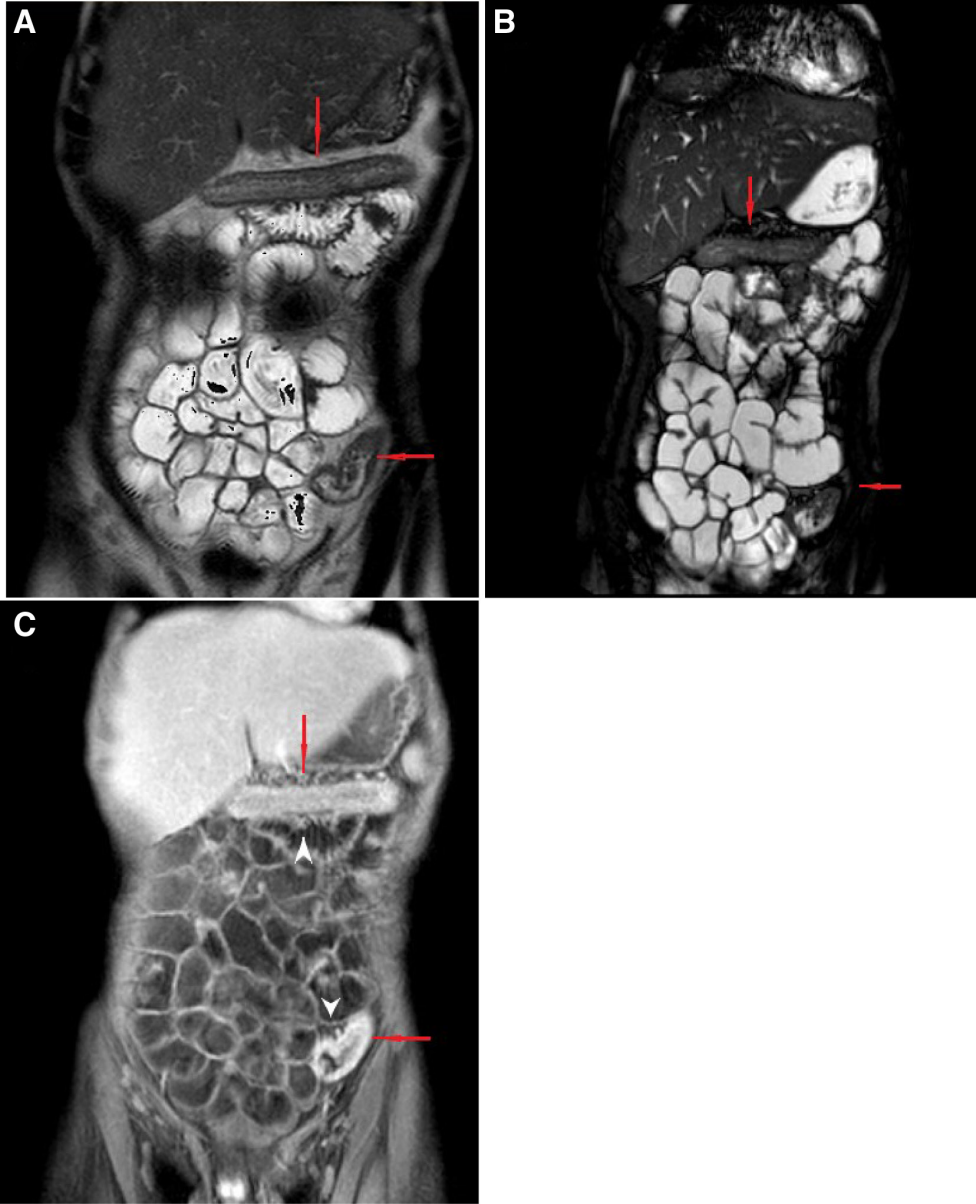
Typical pathological findings of active inflammation in Crohn disease are identified on magnetic resonance enterography with oral polyethylene glycol. A and B) Depiction of wall thickening of the transverse and sigmoid colon (red arrows) on SSFP (A) and SSFSE (B) sequences; (C) Bowel wall enhancement (red arrows) with mesenteric fat thickening and “comb sign” (arrowheads) due to vascular engorgement of mesenteric vessels displayed on gadolinium-enhanced FSPGR sequence. FSPGR = fat-suppressed 3D spoiled gradient echo; SSFP = steady-state free precession; SSFSE = T2-weighted single-shot fast spin-echo.
